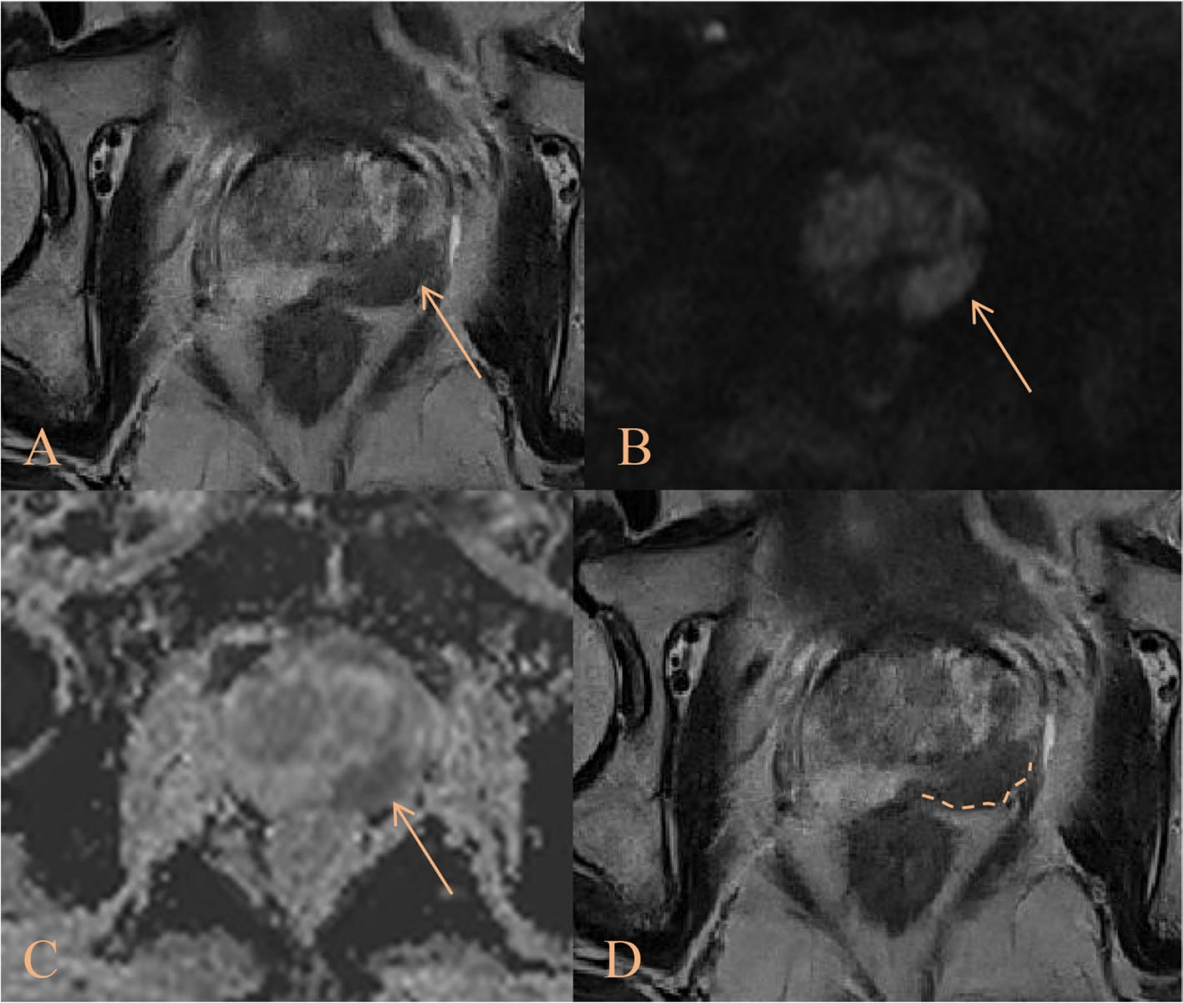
The patient was a 69-year-old male. The A-C images consisted of T2-weighted imaging, diffusion weighted imaging, and apparent diffusion coefficient maps, respectively, with the tumor indicated by the yellow arrow. The D image represented a T2WI image with focal labeling processing, where the curvilinear contact length (CCL) was highlighted by the dashed line. Clinical, radiological and pathological data were as follows: PSA 29.53, PSAD 0.91, CCL 28.2, CCL/LD 1.48, NCI_EPE grade 2, ISUP_GG 4. According to the scoring system, the final value was 7 points, which was divided into intermediate-high tier, and the final pathological result was pT3
